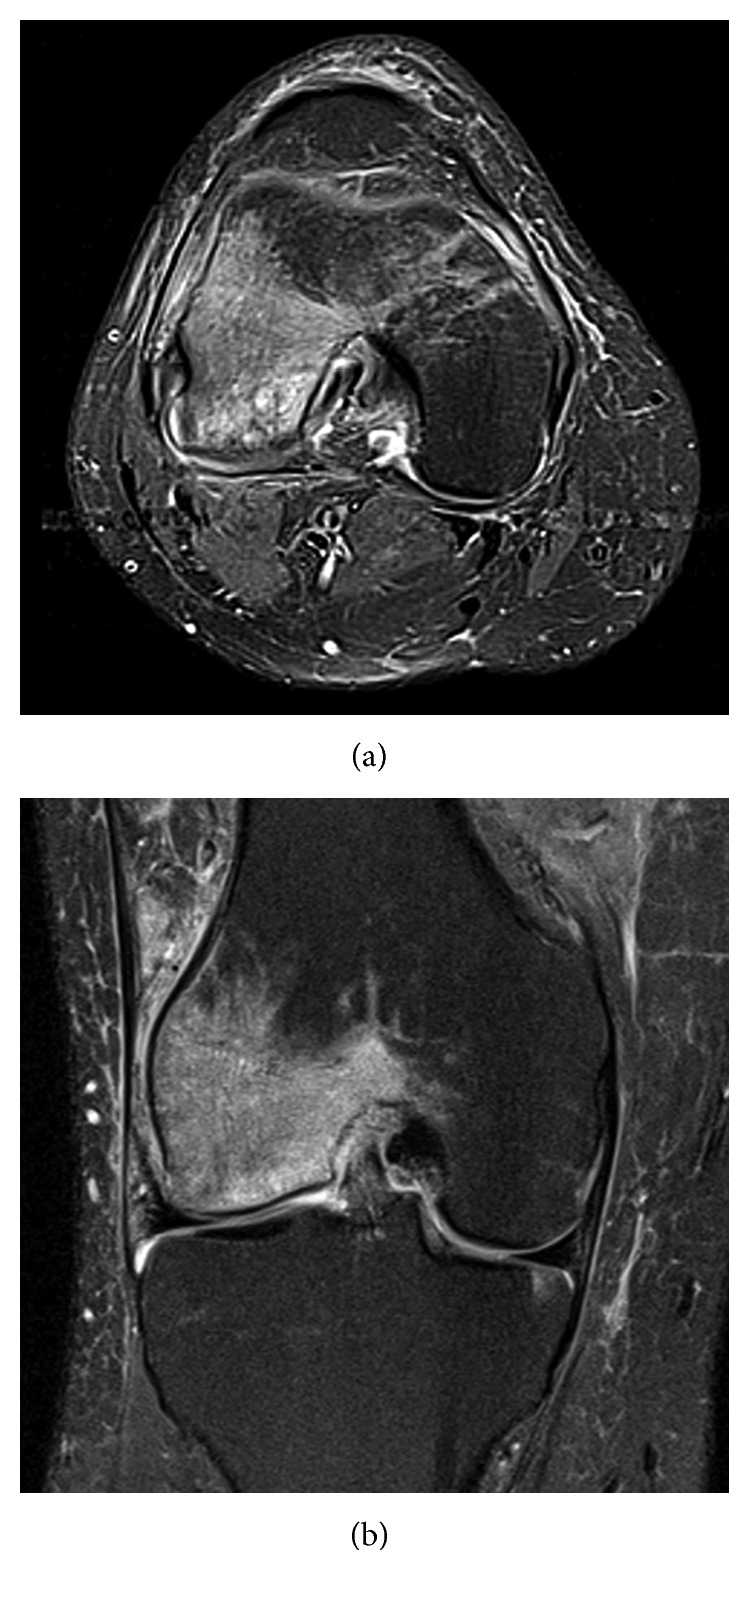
MRI (T2 Fat Sat; (a) axial and (b) coronal views) showing lateral and medial femoral bone oedema (osteochondral collapse of lateral femoral condyle).
